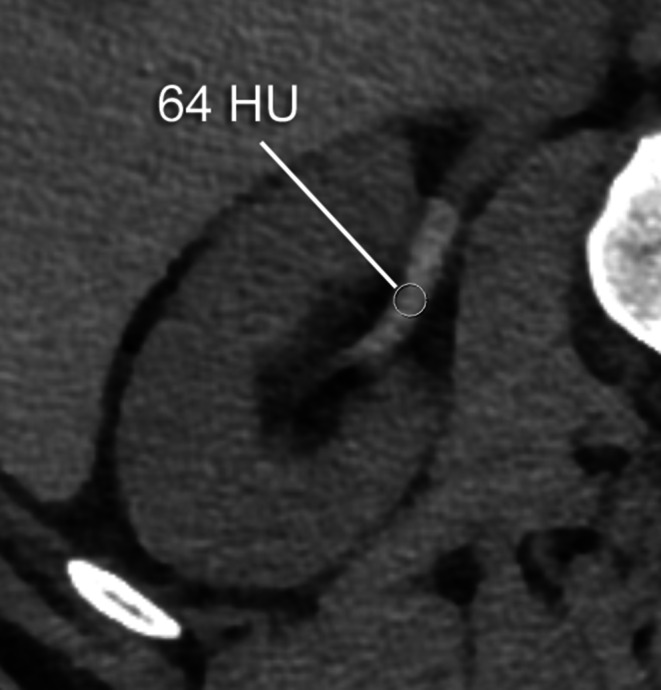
Transversal PMCT after previous intrathecal contrast agent application in a 55-year-old patient with orthostatic headache without epidural CSF collection (SLEC(−)) demonstrating opacification of the renal pelvis and a density measurement using a circular ROI with a mean of 64 Hounsfield units. *CSF* cerebrospinal fluid, *PMCT* postmyelography computed tomography,* ROI* region of interest
